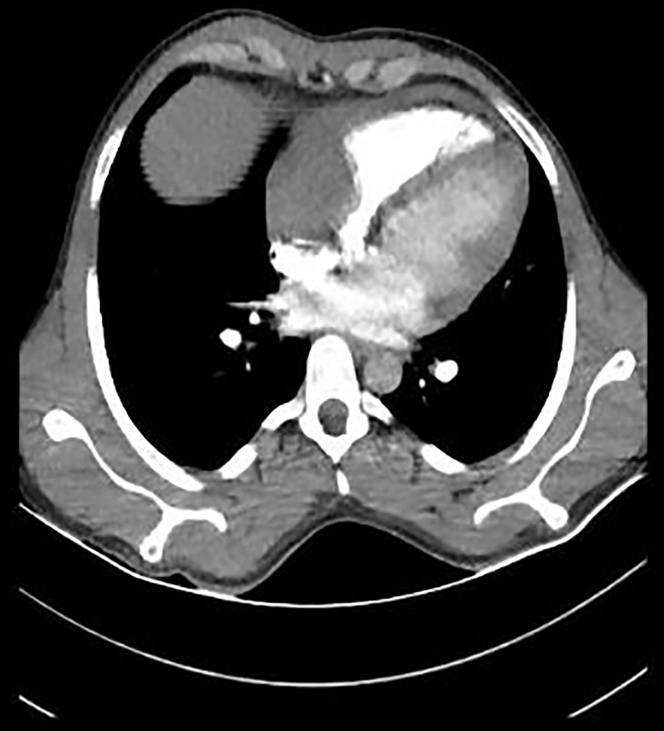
Pulmonary Computed Tomographic Angiography Shows a Space-Occupying Lesion That Largely Fills the Right Atrium and Partially Compresses the Right Ventricle
